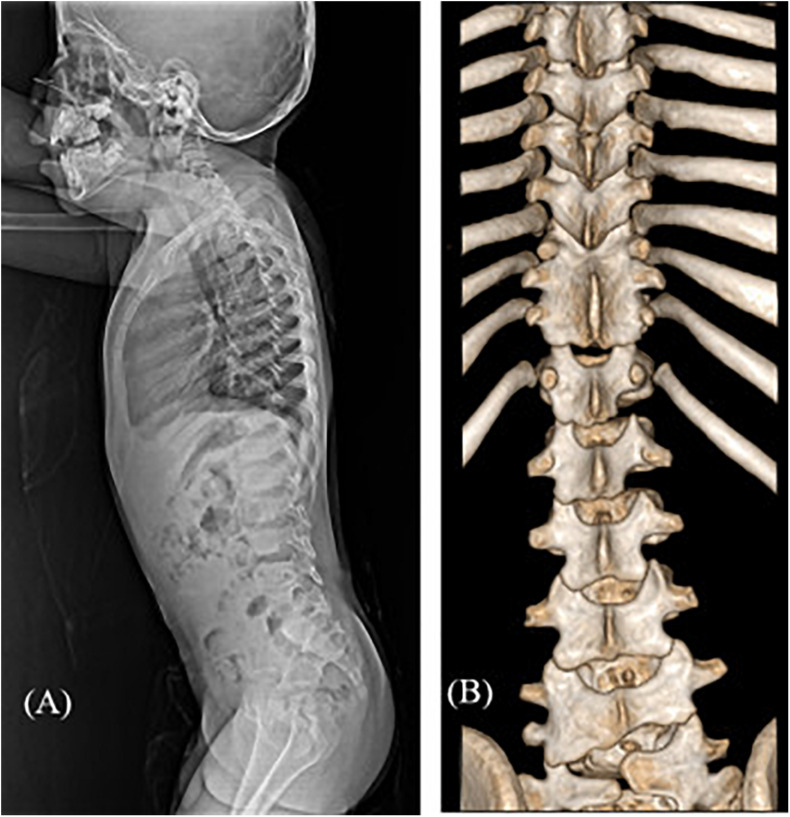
Radiographic images of case #16 with spondylocarpotarsal fusion syndrome 1A. (A) Lateral spine X-ray showing mildly narrowed disk spaces (B) 3D reconstruction CT scan showing fusion of T9-10-11 bilateral facet joints and spinous processes and L4-5 left facet joints.
